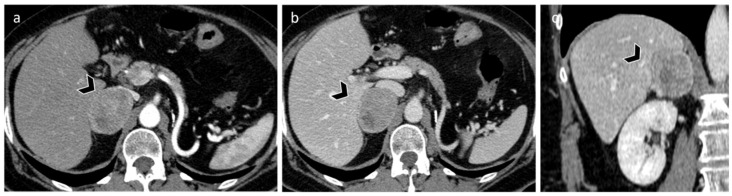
A 65-year-old female with right adrenal pheochromocytoma. CT axial arterial (a) and portal venous phases (b,c) show inhomogeneous adrenal solid lesion with strong and heterogeneous enhancement; the lesion showed an absolute wash-out of 77%.
